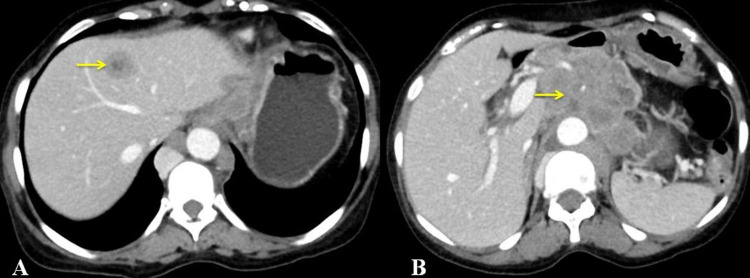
Contrast-enhanced computed tomography (axial view) showing (A) metastatic lesion in the liver suggested by target lesion (arrow) and (B) confluent large heterogeneously enhancing lymph nodal mass (arrow).
